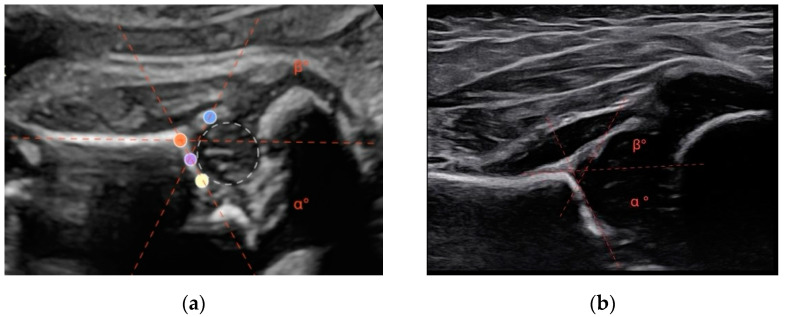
(a) During the ultrasonographic examination of a 34-week-old fetus, the hip near the maternal abdominal wall was visualized. The acetabular roof (orange), the turning point (purple), the lower edge of the ossified ilium (yellow), and the labrum (blue). The angles alpha and beta are determined by the intersection of the lines connecting the specified reference points. In this case, the alpha angle measures 61°, while the beta angle measures 64°. (b) The same patient, in the postnatal ultrasound at 6 weeks of age, shows an alpha angle of 65° and a beta angle of 60°.
